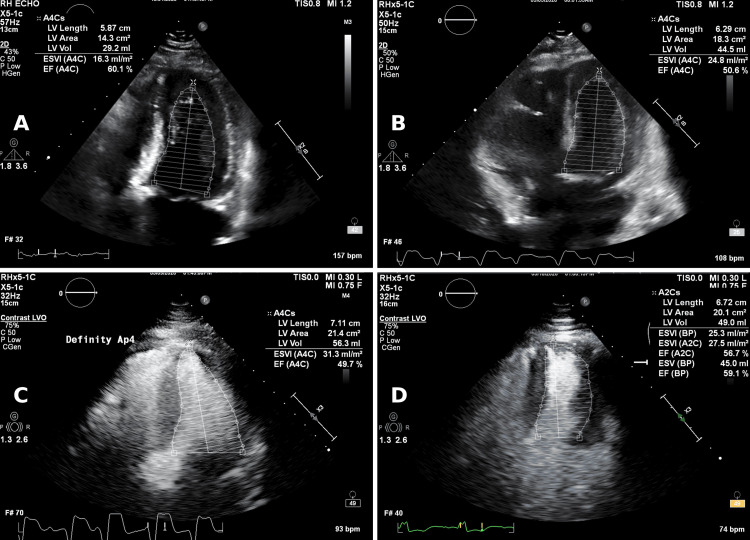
Serial transthoracic echocardiograms demonstrating left ventricular ejection fraction during pembrolizumab-induced MMM overlap syndrome. (A) Baseline echocardiogram (four months prior) showing normal systolic function, with an LVEF of 60%. (B) Echocardiogram on day 3 demonstrating a decline in systolic function to LVEF 48%. (C) Definity contrast-enhanced study on day 7 confirming continued mild systolic dysfunction, with an LVEF of 45%. (D) Follow-up Definity contrast-enhanced echocardiogram on day 16 demonstrating recovery of systolic function, with an LVEF of 59% after immunosuppressive therapy.
